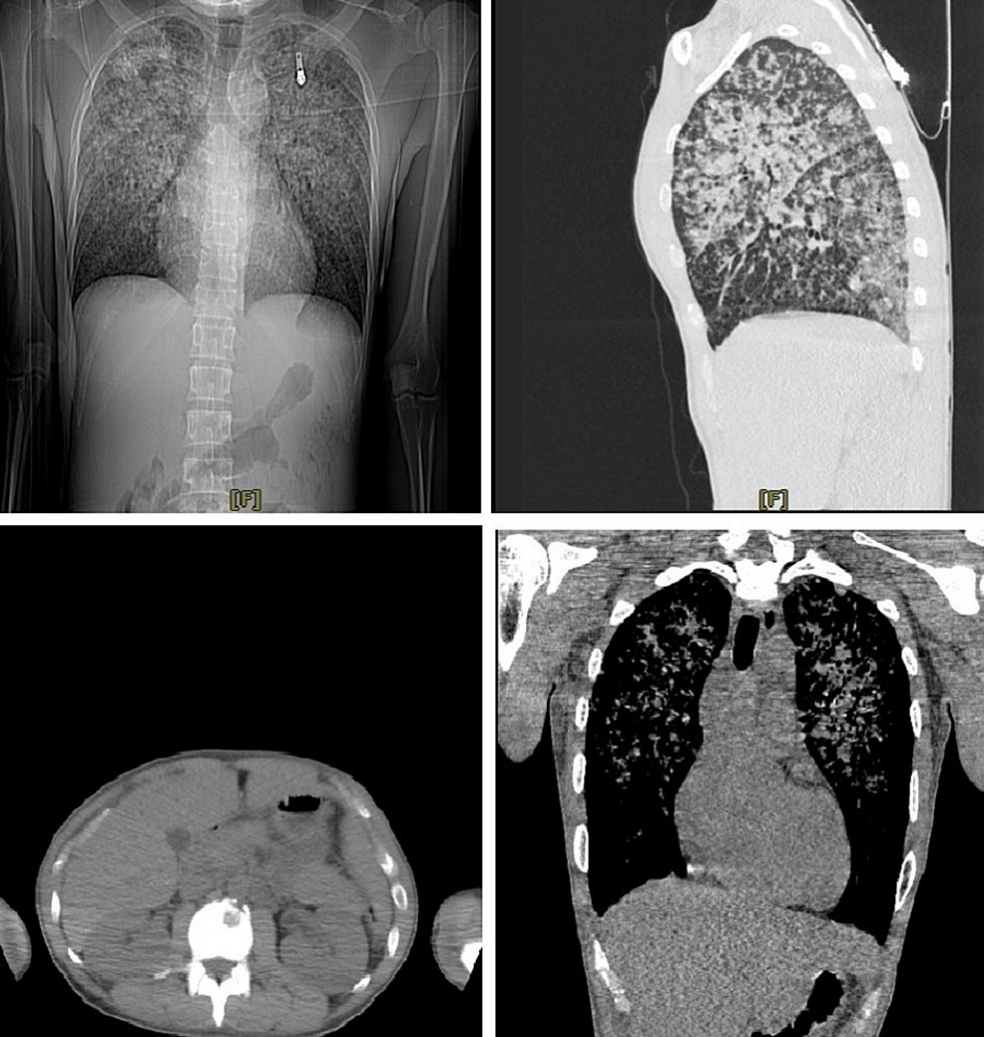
CT scan of the chest as seen from different angles. Numerous bronchovascular distribution of nodules, worse in upper central lungs, can be observed. An osteolytic lesion can also be seen in the lower left quadrant.
